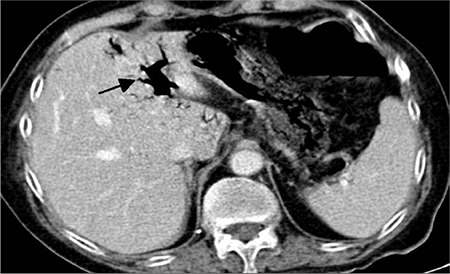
Contrast-enhanced abdominal computed tomography demonstrates air in portal vein (black arrow).
